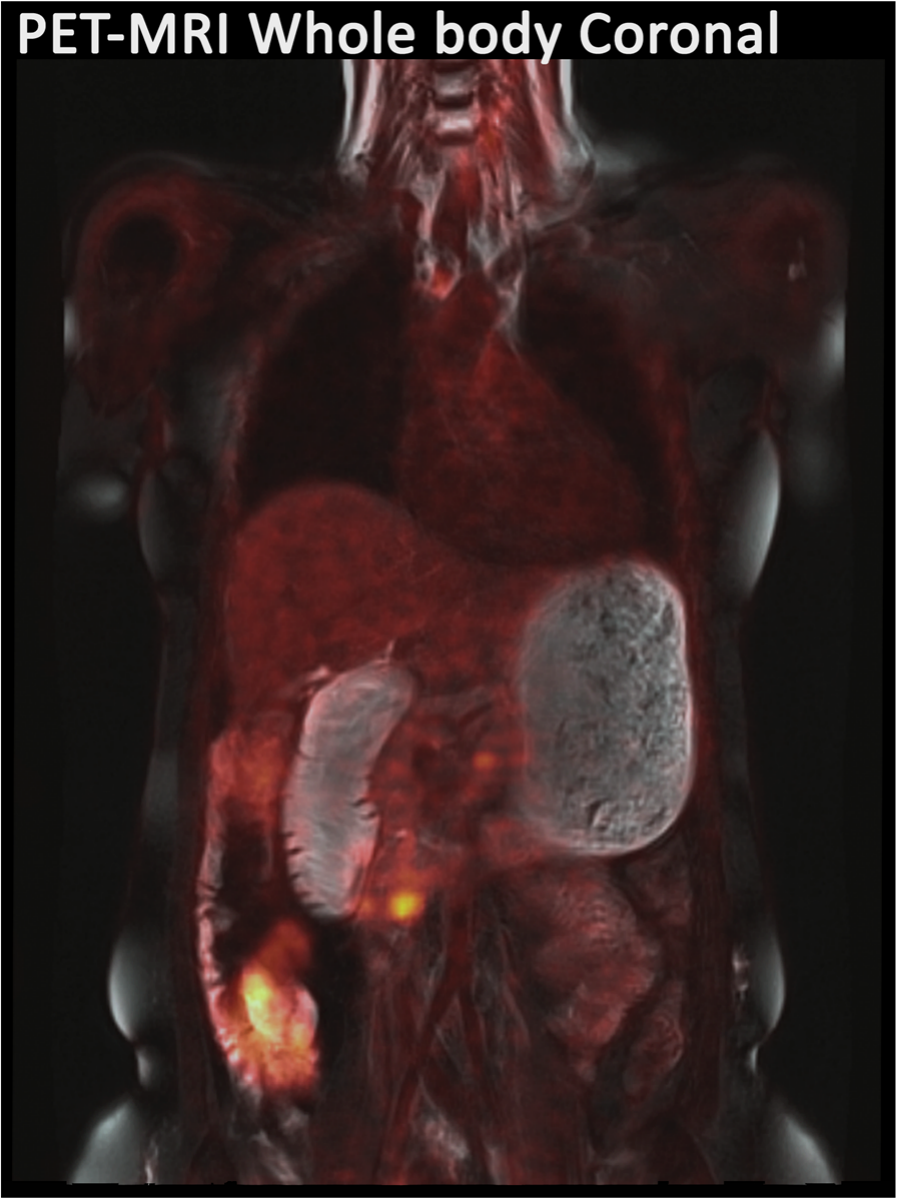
Whole-body PET-MRI fusion showing para-aortic lymph node metastasis
